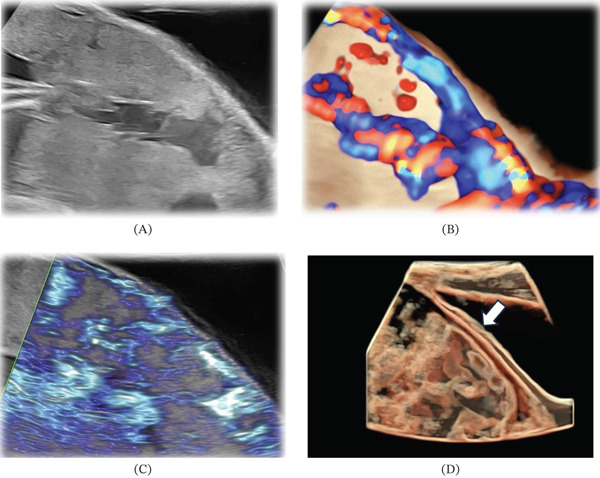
Ultrasound scan shows the placenta located at the anterior wall of the lower uterine segment, covering the internal os of the cervix. (A) Gray‐scale ultrasound signs reveal placental bulge, myometrial thinning, loss of “clear zone,” abnormal lacunae, and bladder wall interruption. Neovascularization signs, such as bridging vessels and “rail signs,” are detected with (B) color Doppler ultrasound and (C) power Doppler ultrasound. (D) Tramline disruption (white arrow) is observed on three‐dimensional ultrasound.
